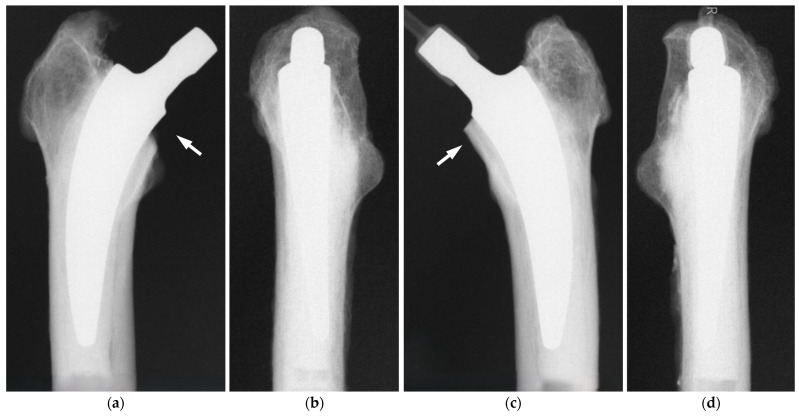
Anteroposterior and lateral radiographs of a pair of femora (individual XV, see Table 1) after implantation of the cemented short stem A2 (ARTIQO GmbH, Lüdinghausen, Germany) with the low resection in the right femur (a,b) and the high resection in the left femur (c,d), indicated by white arrows.
